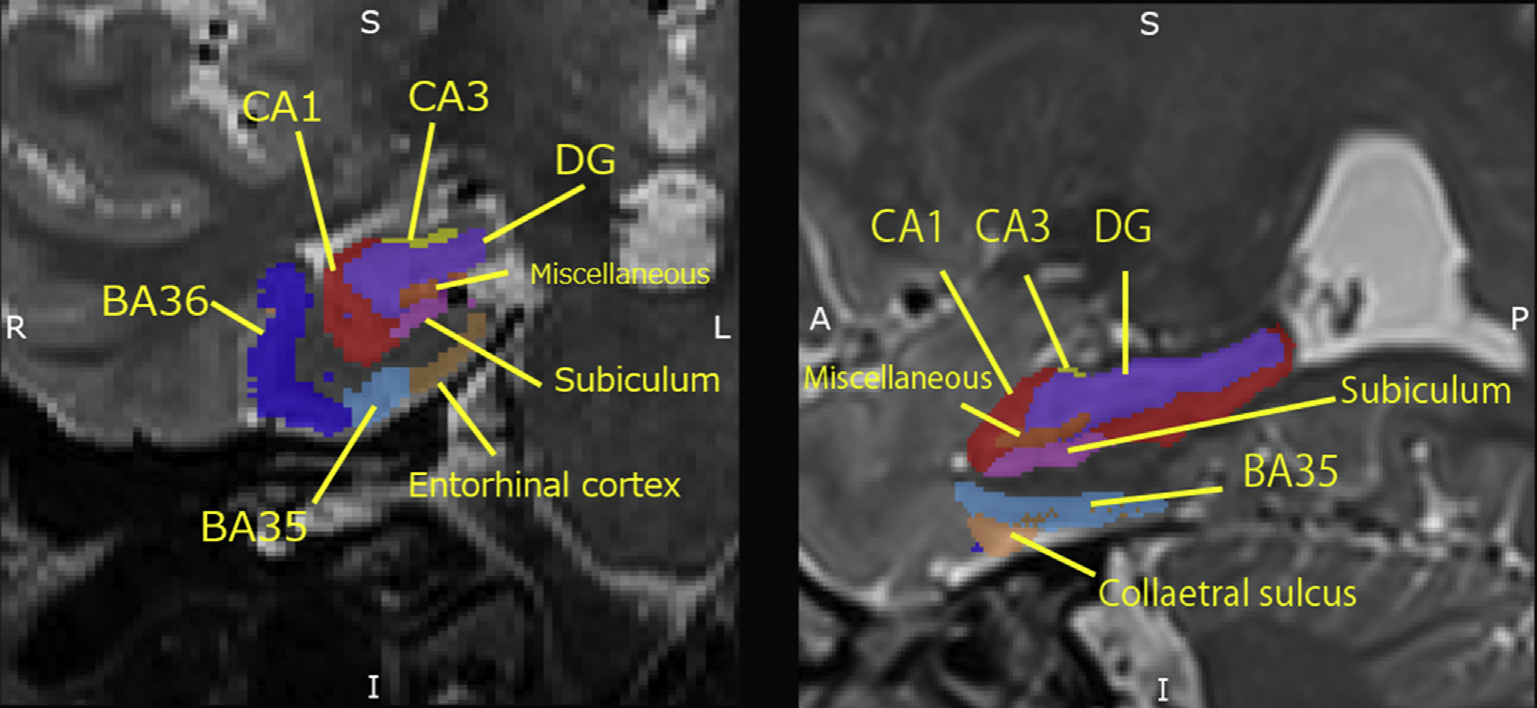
Automatically segmented subfield areas of the medial temporal lobe on high-resolution T2-weighted coronal (left) and sagittal (right) images.
